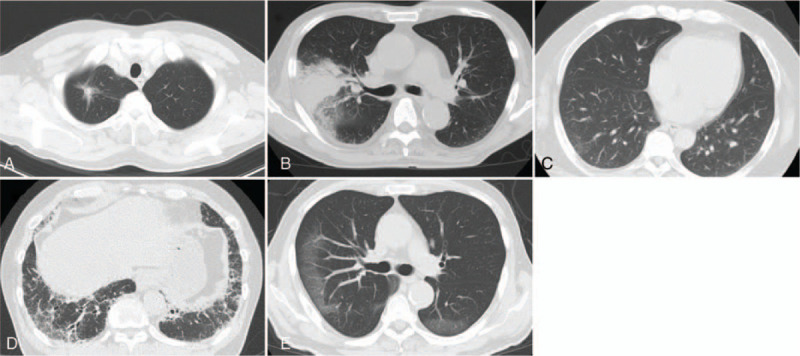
Representative features of chest computed tomography (CT) classifications. Chest CT scans of outpatients with fever. CT features classified into 5 categories according to the COVID-19 Reporting and Data System (CO-RADS). (A) CO-RADS 1, normal CT or non-infectious etiology (e.g., lung tumor, lung fibrosis). (B) CO-RADS 2, infectious etiology that is not compatible with COVID-19 (e.g., infectious bronchiolitis, bronchopneumonia, lobar pneumonia). (C) CO-RADS 3, equivocal findings for COVID-19 (e.g., homogeneous extensive ground-glass opacities [arrow]). (D) CO-RADS 4, typical CT findings of COVID-19 (similar to CO-RADS 5) but showing some overlap with other pneumonia. (E) CO-RADS 5, typical CT findings of COVID-19 (e.g., multifocal ground-glass opacities with or without consolidation in lung regions close to visceral pleural surfaces).
